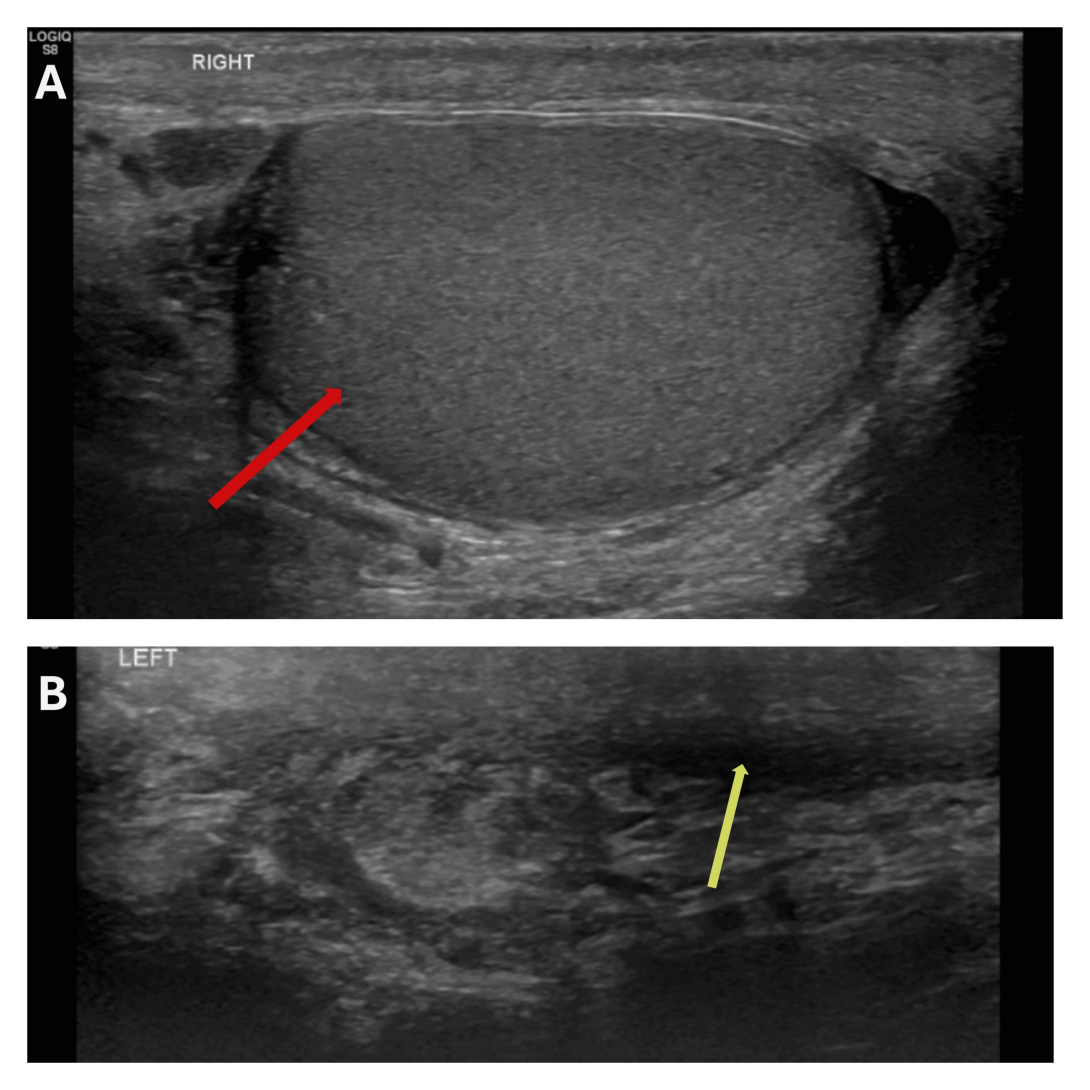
Six-month follow-up scrotal ultrasound showing (A) a normal right testicle (red arrow) and (B) a left empty hemiscrotum with no local recurrence (yellow arrow).
